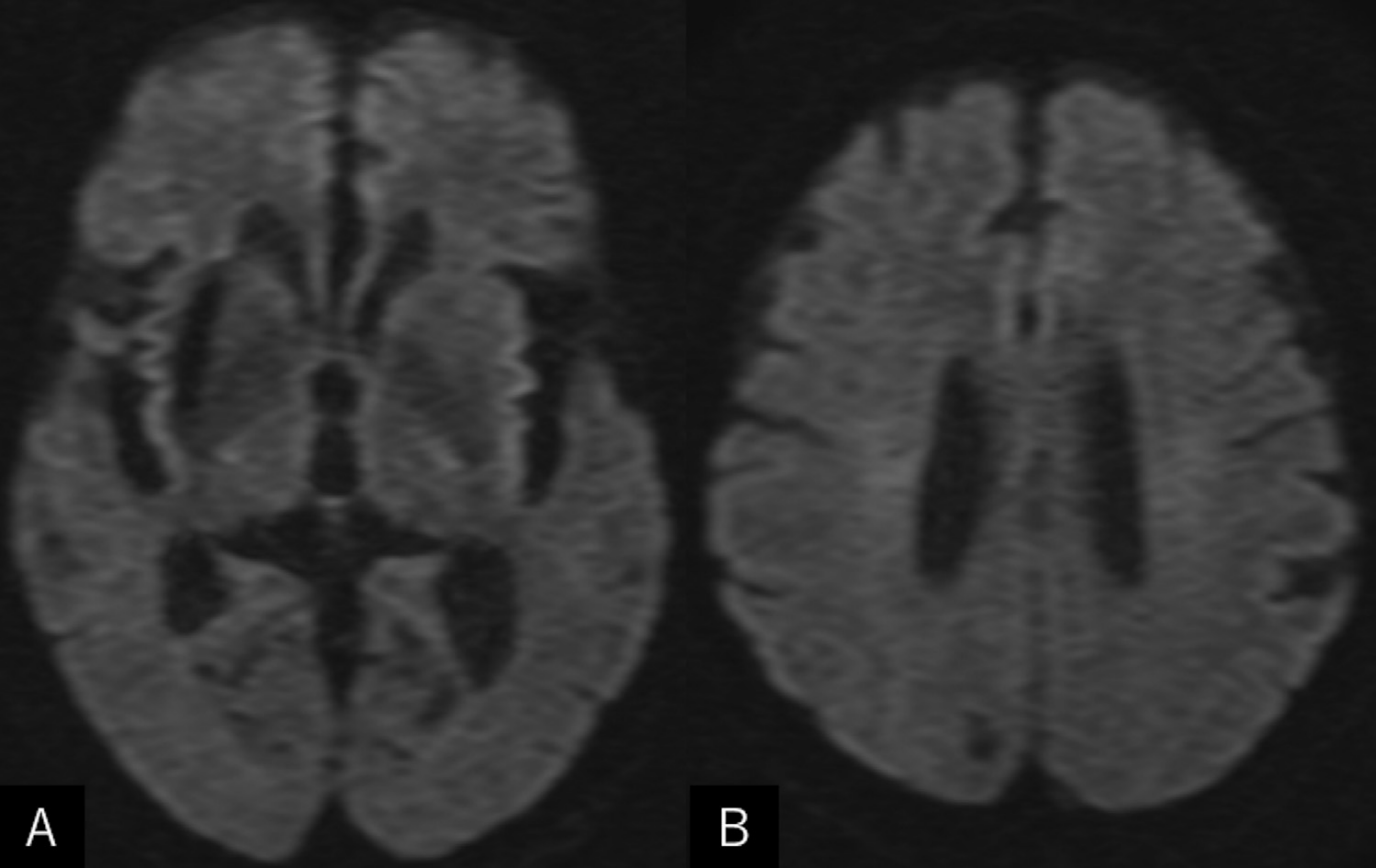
(*A, B*) Diffusion-weighted magnetic resonance images showing absence of infarcts in super-acute phase.
